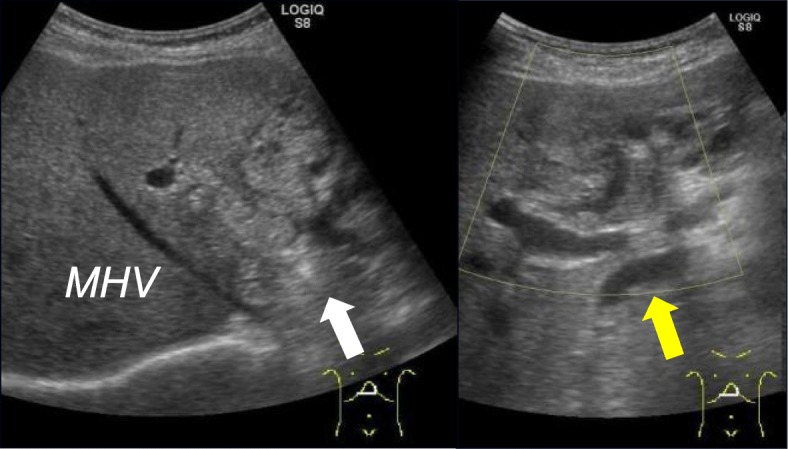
Preoperative ultrasonography. Abdominal ultrasonography showed a hyperechoic lesion, 40 mm in diameter, located in the left hepatic bile duct (white arrow), and extended dilation of the distal left intrahepatic bile duct (yellow arrow)
